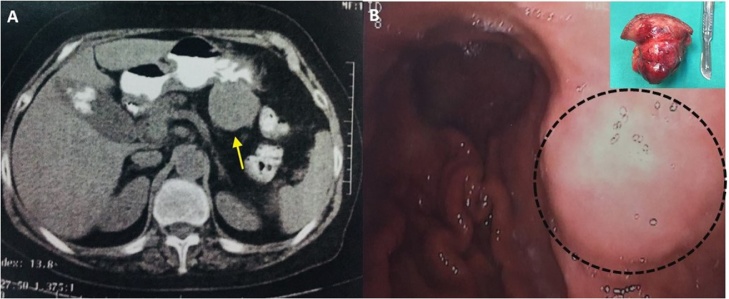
Contrast enhanced CT showing a round, well-defined and homogeneous gastric mass (Fig. 4A) and endoscopic detection (Fig. 4B) in submucosal of the greater curvature of the stomach. In the frame, macroscopic view of the resected mass shows a large exophytic mass along the less curvature of the stomach.
